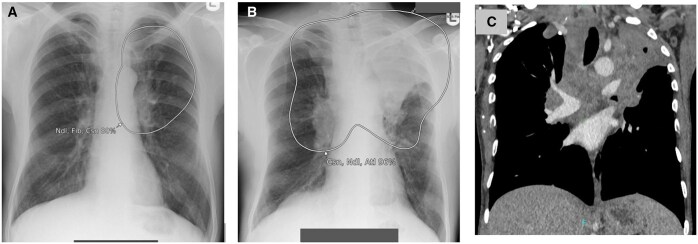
A 51-year-old male with a history of smoking, weight loss, and haemoptysis underwent a CXR (A), reported as NAD. The AI software demonstrates suspicion of a nodule in the left paramediastinal region. Ten months later, the patient returns for another CXR (B) due to persisting cough and chest pain. Both the report and AI confirm suspicion of an underlying central tumour. A subsequent CT was performed (C) demonstrating a large central left-sided mass, confirmed to be stage IV LC. AI = artificial intelligence; CXR = chest radiograph; LC = lung cancer; NAD = no abnormility detected.
